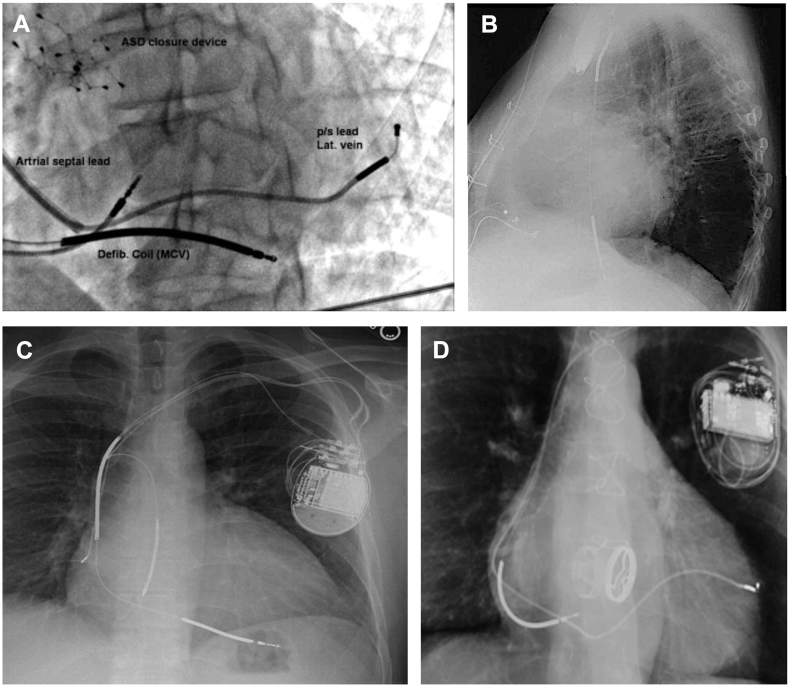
Different approaches to prevalvular transvenous ICD implantation. A: ICD in MCV and pace/sense lead in posterolateral vein of CS (Adapted from Elsevier; Source: Lopez et al, 2009^55^); B: Floating ICD in IVC visible on lateral chest radiograph (Adapted from John Wiley and Sons; Source: Schreiber et al, 2003^56^); C: Additional ICD in azygous vein (pictured along the spine) on postero-anterior chest radiograph (Adapted from Elsevier; Source: Cooper et al, 2009^61^); D: ICD in low right atrium with coronary sinus lead in posterior branch of CS (Adapted from John Wiley and Sons; Source: Biffi et al, 2006^57^).
